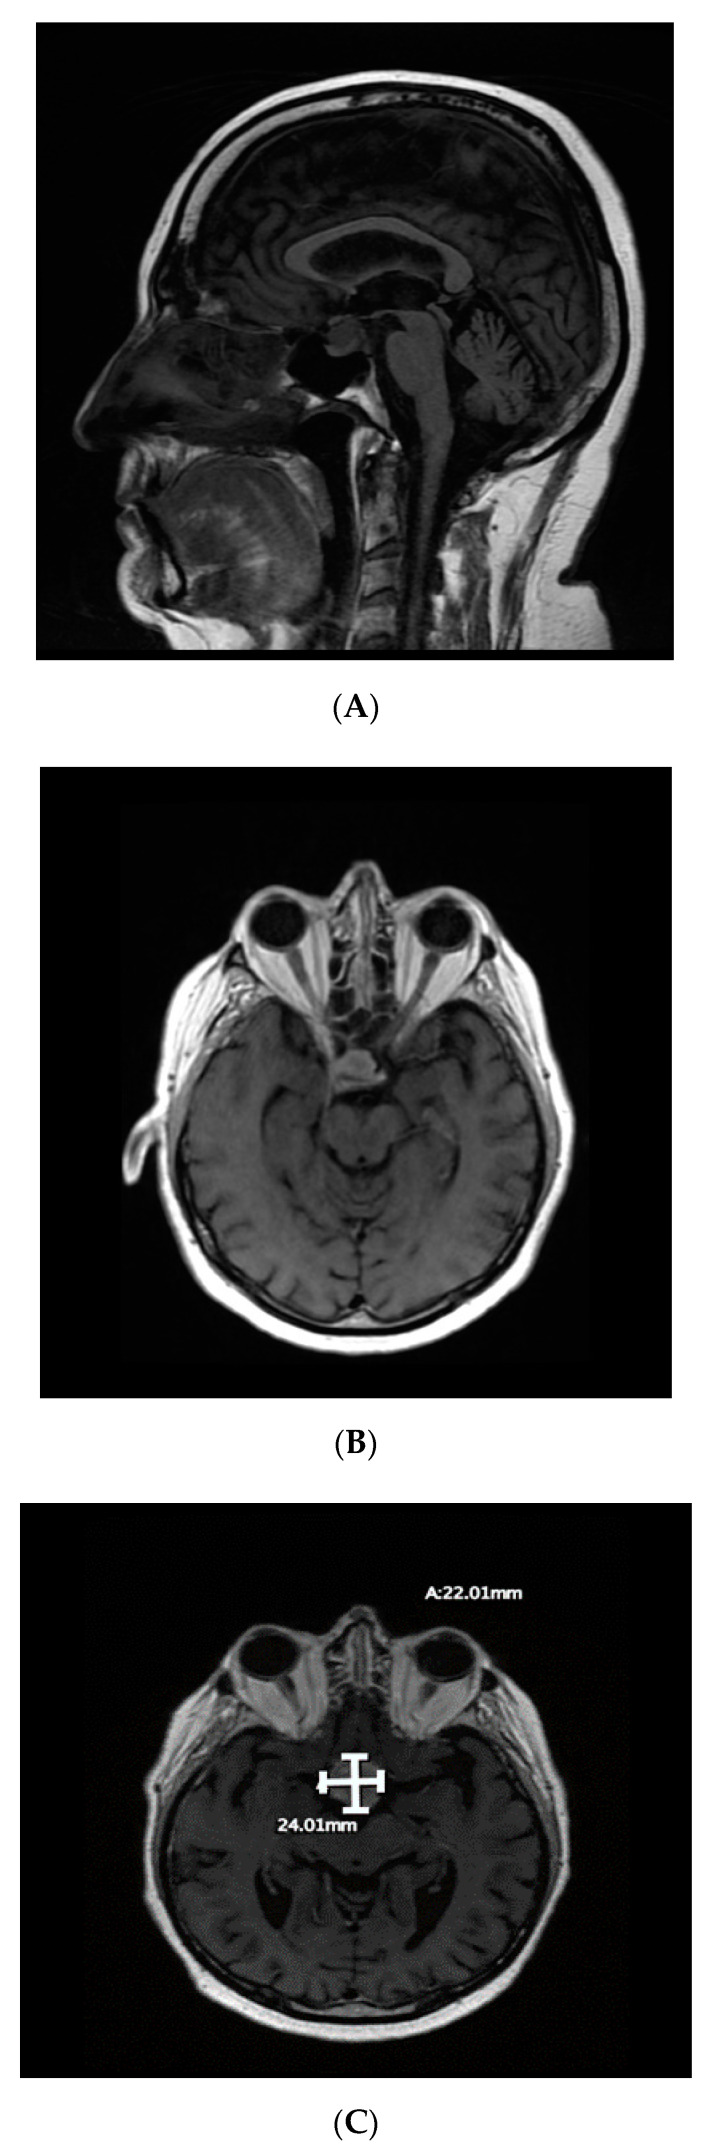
(A,B) Preoperative magnetic resonance imaging of the brain (June 2018) revealing a right sellar mass approximately 2.12 × 1.4 × 1.2 cm in size (A: sagittal view; B: axial view); (C) imaging during surgery in September 2018 showed that the mass had increased in size.
